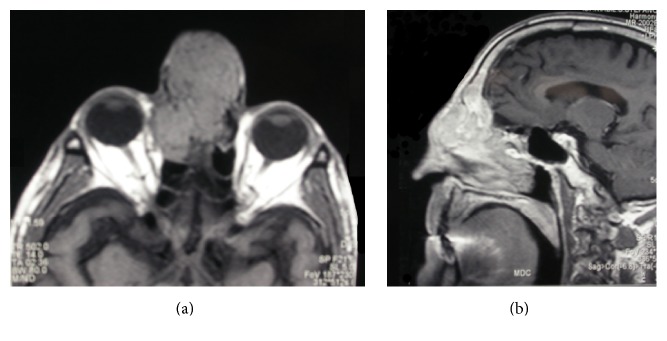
MRI in the axial (a) and sagittal (b) planes showing a soft tissue ethmoid mass extending to the right and left choanal region, the right orbit, the right frontal sinus, and an initial intracranial extension with partial erosion of the crista galli.
